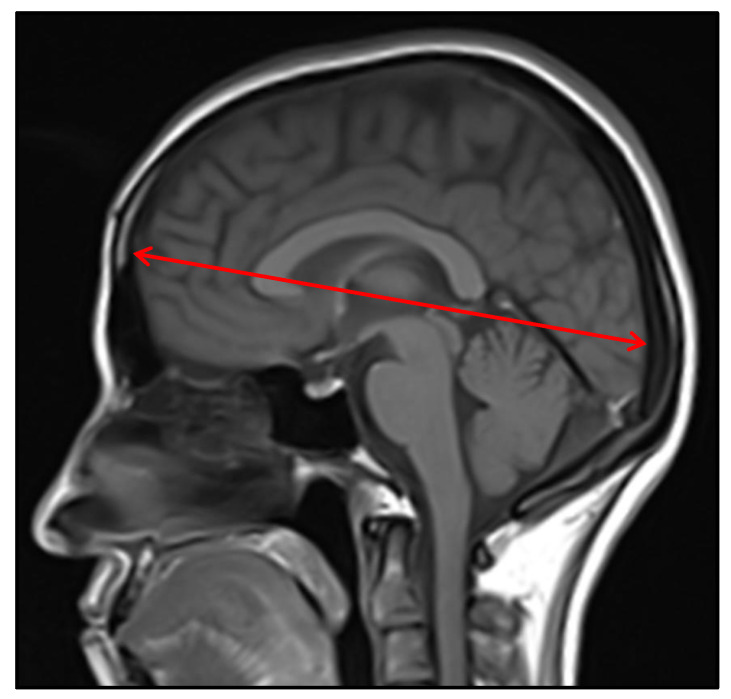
Fronto-occipital length measurement on midsagittal T1W MRI image.
